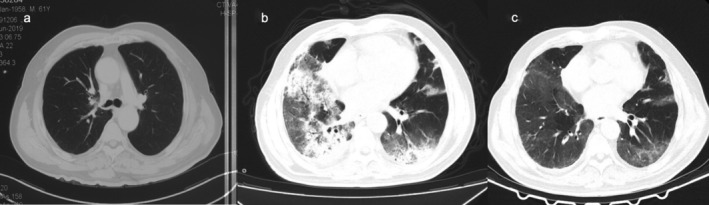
Computed tomography (CT) scan findings in Case 2. (a) Stable disease after six cycles of paclitaxel, carboplatin and pembrolizumab. (b) Extensive ground‐glass opacities (GGOs) and multifocal consolidations were evident. (c) Radiographic lesions resolved markedly after antimicrobial and glucocorticoid treatment.
